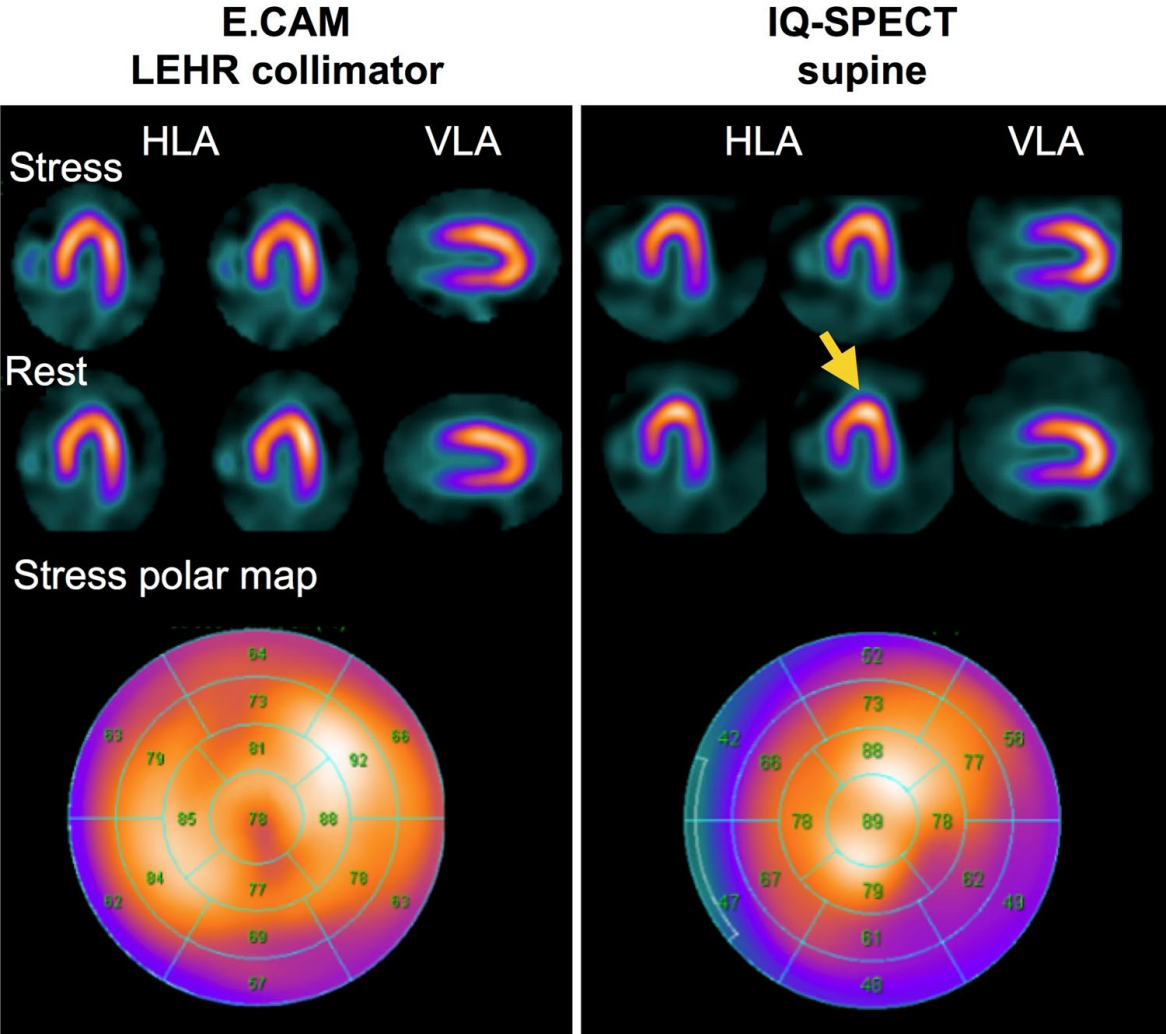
Comparison of conventional MPI (E.CAM, Siemens) in the supine position and IQ·SPECT. IQ·SPECT images and polar map show hot apex (arrow) and inferolateral attenuation. *LEHR* low-energy high-resolution, *HLA* horizontal long-axis, *VLA* vertical long-axis
